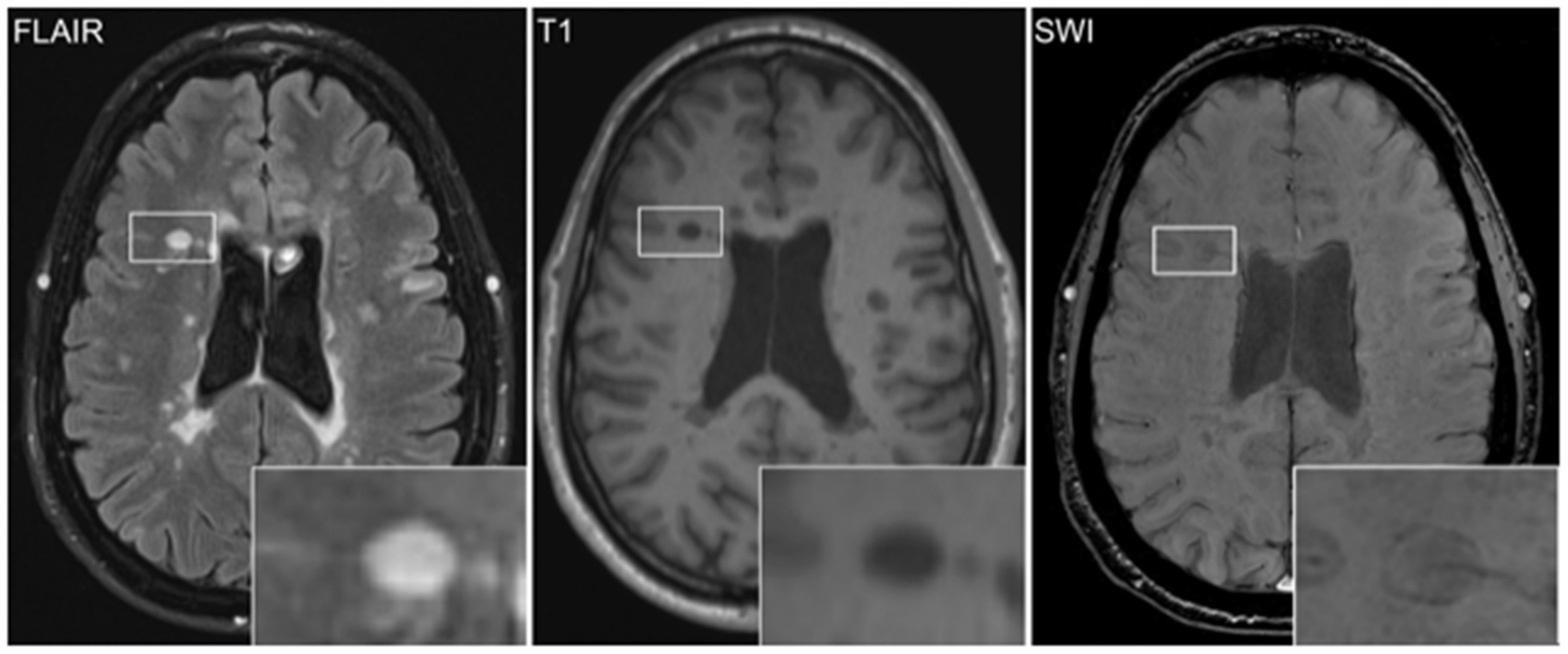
Iron rim lesions (IRLs) can be visualized by MRI *via* susceptibility-weighted imaging (SWI) as a hypointense rim of iron-laden microglia and macrophages surrounding the FLAIR-hyperintense lesion. Increased T1 hypointensity of the IRL indicates severe tissue destruction.
